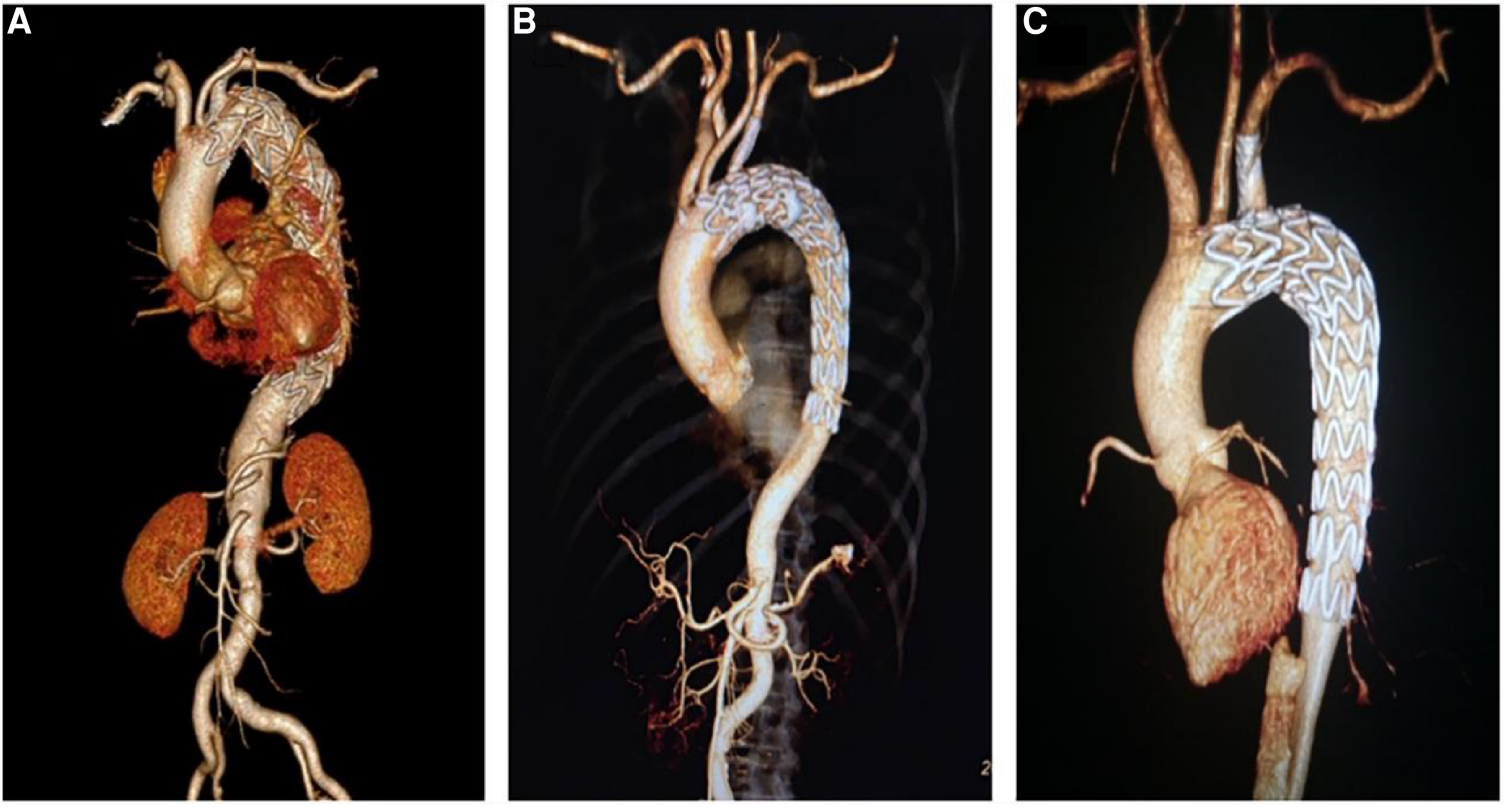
Postoperative computed tomography angiography of three different fenestration methods. (A) Laser fenestration, (B) needle fenestration, (C) quick fenestration.
